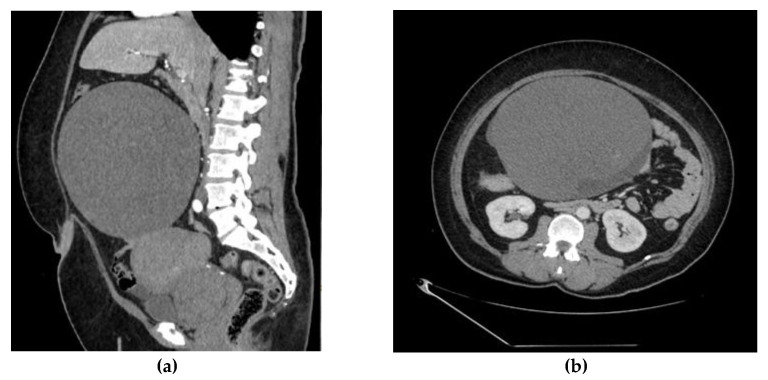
(a). Abdominal CT scan shows enlarged and deformed uterus, large (207 × 155 × 182 mm) thin-walled inhomogeneous tumor, connected to uterus and right ovary. (b). Abdominal CT scan shows multiple uterine myomas and a large (207 × 155 × 182 mm) thin-walled inhomogeneous tumor.
